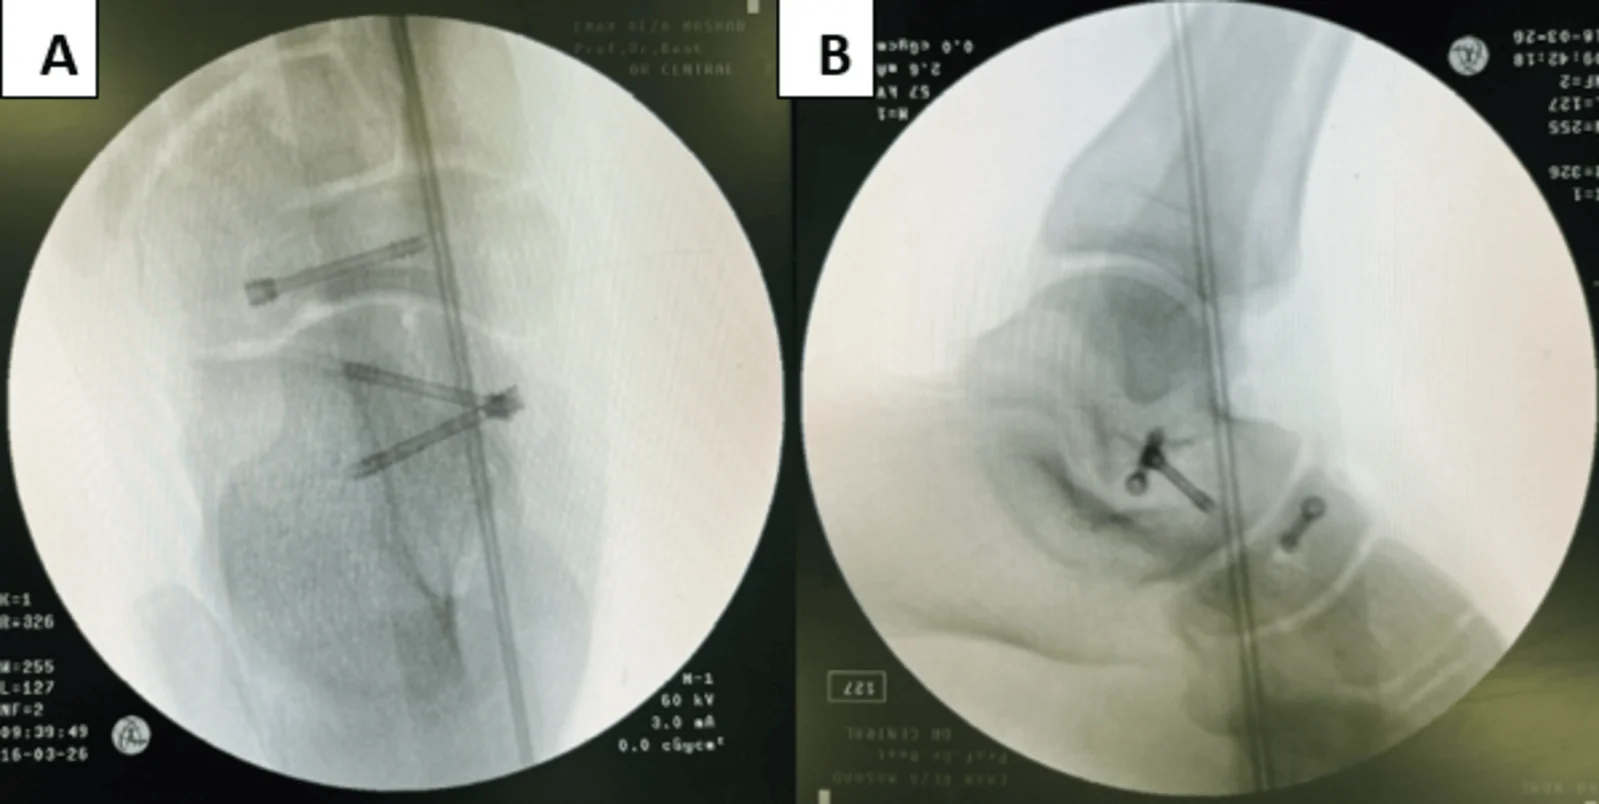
Immediate postoperative radiographs (A) Anteroposterior view and (B) lateral view showing good reduction and fixation
